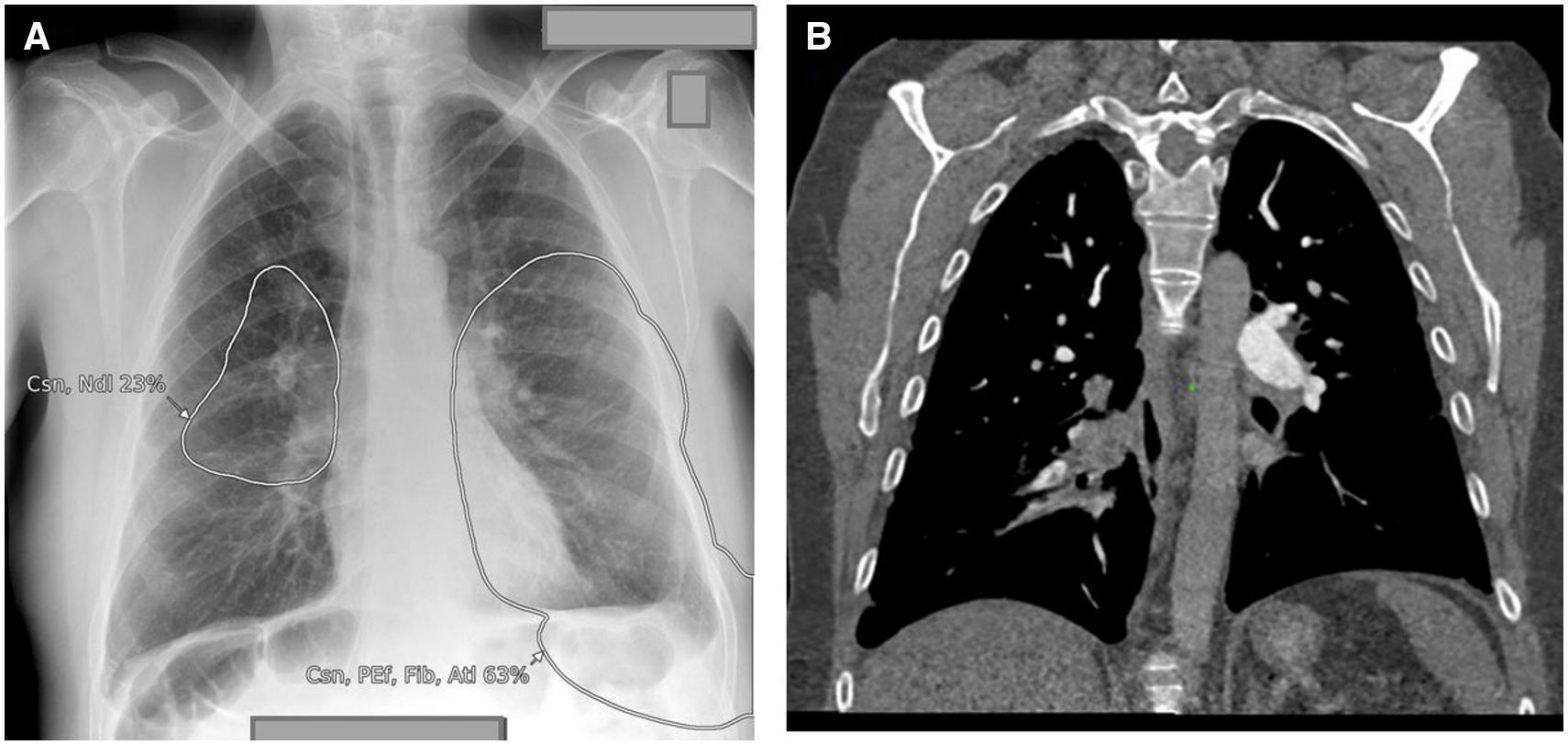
A 55-year-old male smoker with a history of cough underwent a CXR (A) reported as NAD. The AI software demonstrates suspicion of bilateral consolidation with secondary suspicion of a nodule in the right hilar region. Nine months later, a CT scan was performed (B) due to worsening SOB and productive cough, demonstrating the presence of a right hilar mass confirmed to be stage IV LC. AI = artificial intelligence; CXR = chest radiograph; LC = lung cancer; NAD = no abnormility detected; SOB = shortness of breath.
